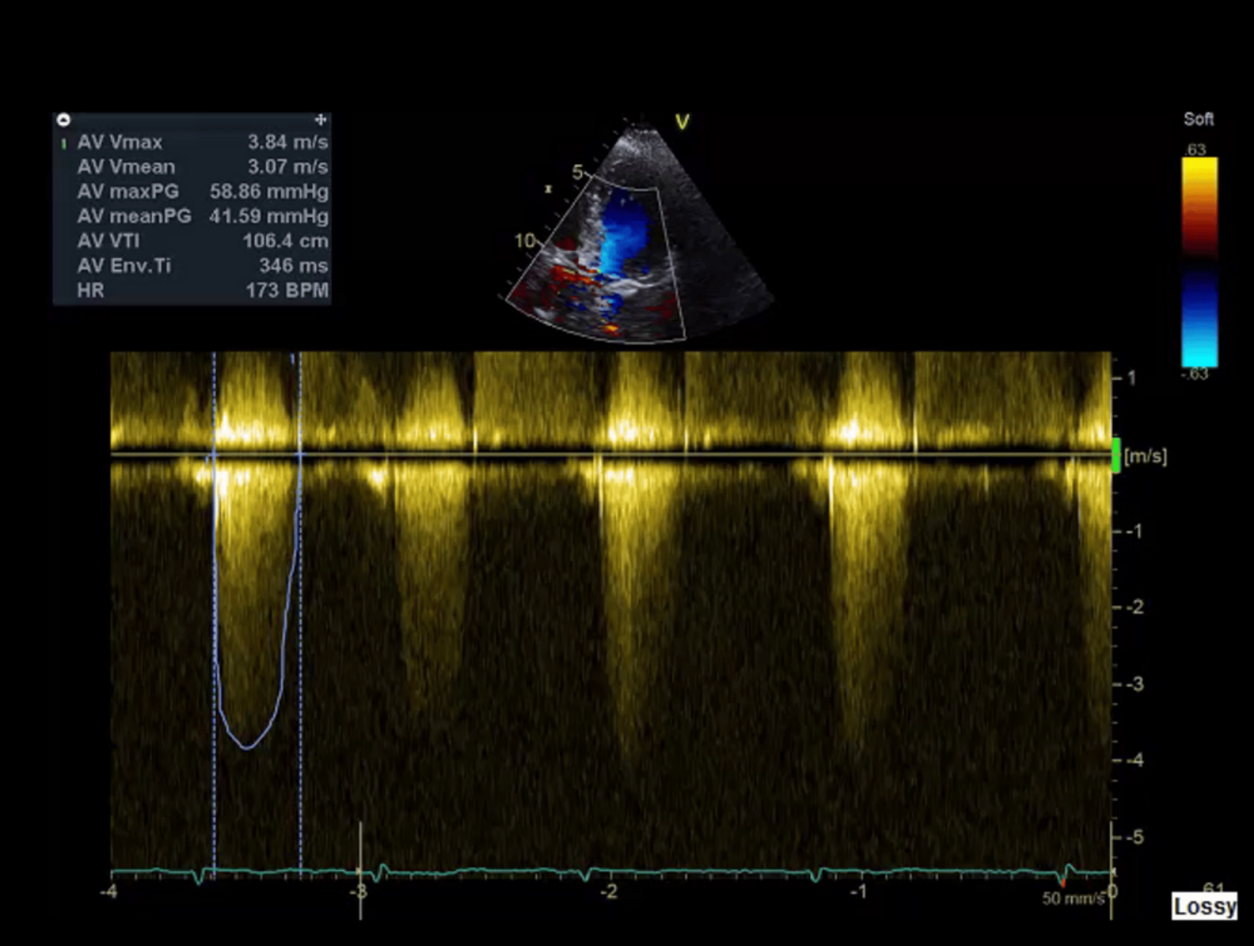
Echocardiograph showing a peak velocity of 3.84 m/s and a mean gradient of >40 mmHg.
